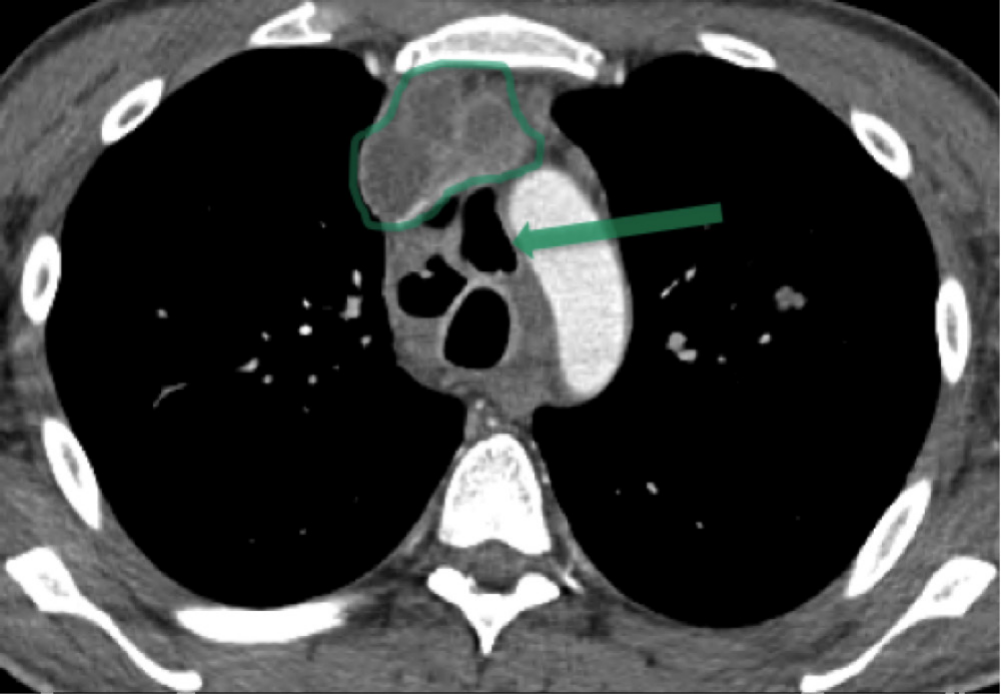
Contrast-enhanced CT scan of the chest on admission revealed multiple low-attenuation necrotic lymph nodes (free-hand-circle) and gas-containing mediastinal collection, representing a mediastinal abscess (arrow).
